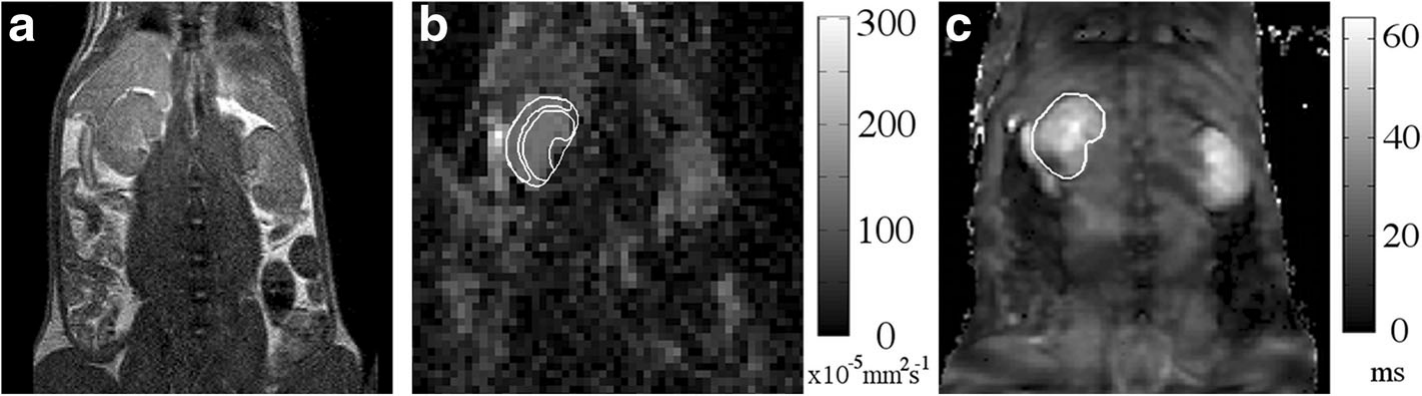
Representative MRI images. Typical images from a) T_2_-weighted anatomical scan, with corresponding b) ADC map and c) T_2_* map from a matched slice, including example ROIs to indicate the selections for analysis of renal cortex and medulla
